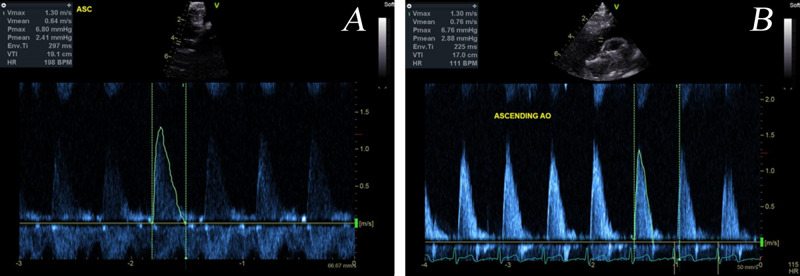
Example Doppler echocardiography dataset taken at the ascending aorta pre- (A) and post-repair (B). The velocity time plot that was digitized is outlined.
